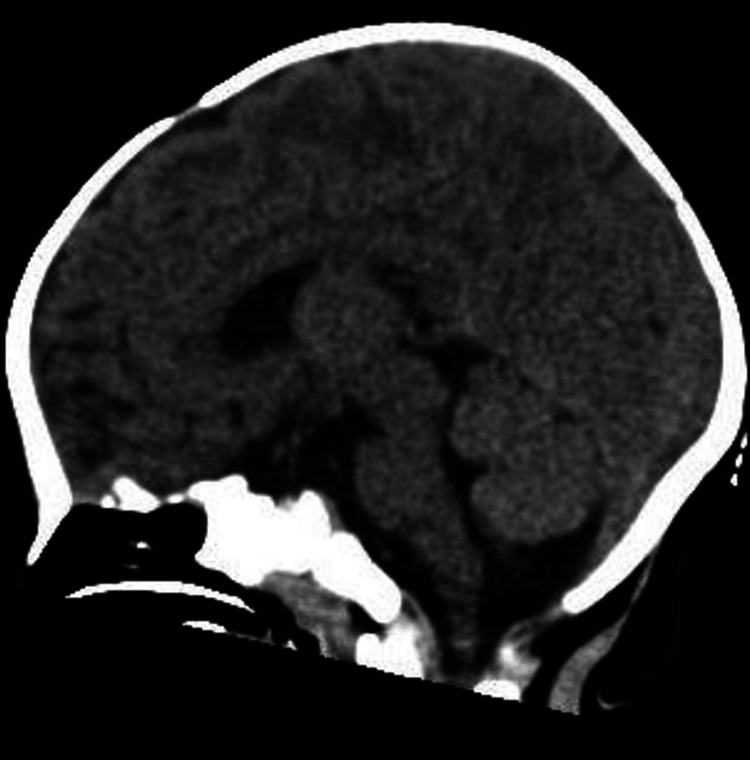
Sagittal view of the brain CT scan displaying no pathological findings.
